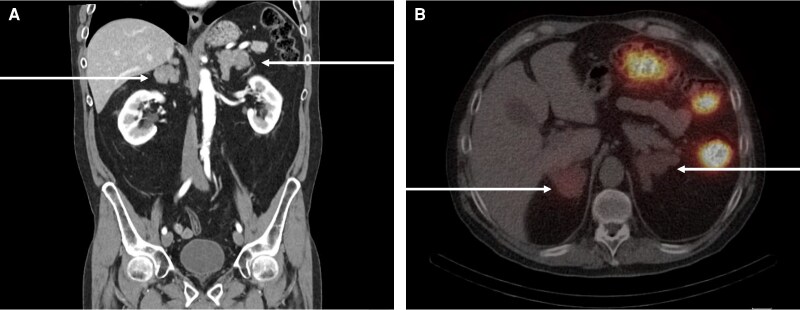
A, Computed tomography of the abdomen showing the massively enlarged bilateral adrenal glands. B, Nor-cholesterol scintigraphy revealed a greater uptake in the right adrenal gland.
